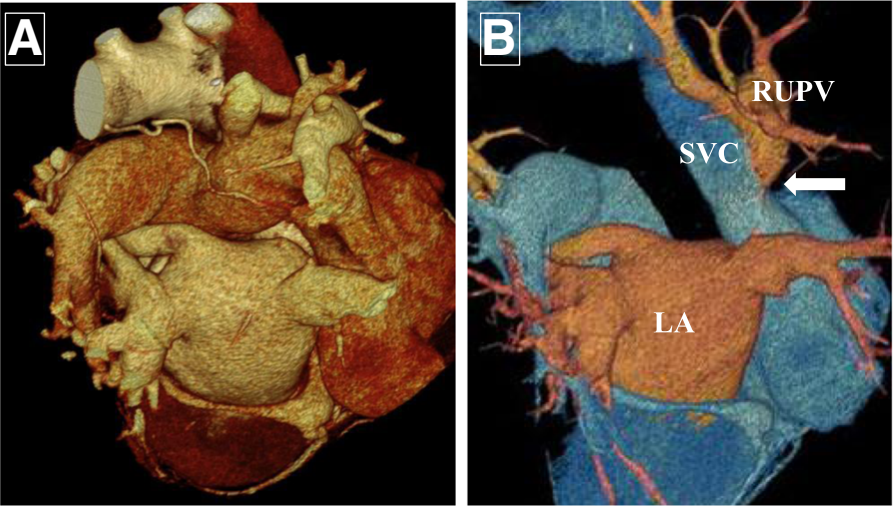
Preoperative three-dimensional computed tomography findings. a Dorsal view. b Image excluding right pulmonary artery. *Arrow* indicates where right upper pulmonary vein (RUPV) connects to the superior vena cava (SVC) rather than the left atrium (LA)
